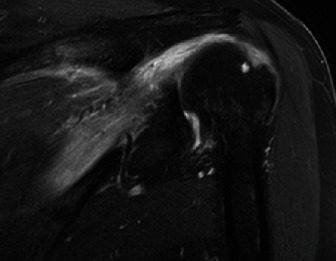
Coronal T2 MRI of the left shoulder demonstrating hyperintensity throughout the infraspinatus muscle, consistent with myositis.
